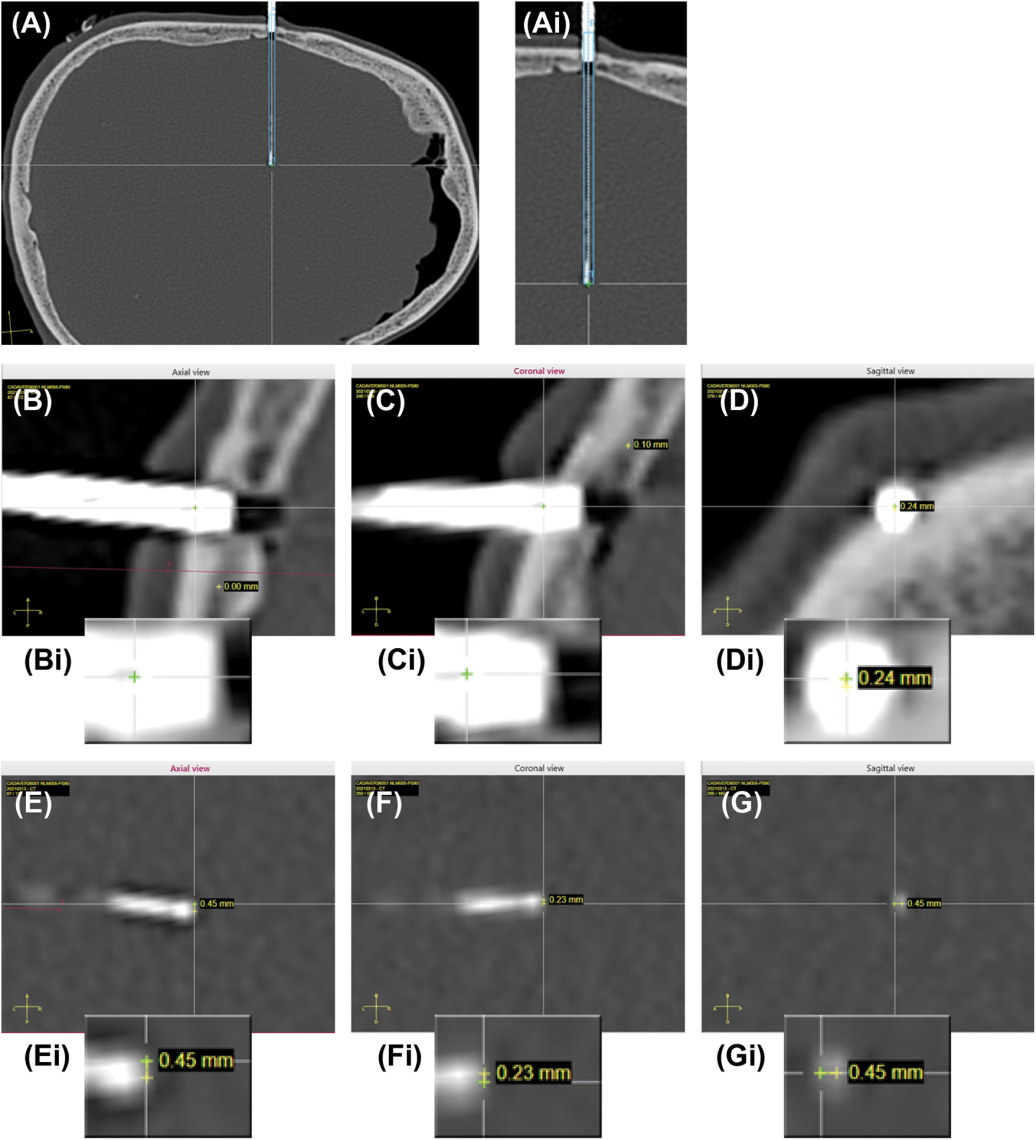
Planned and actual trajectories. (A) CT scan image of an actual implanted electrode is co-registered with the planned trajectory (dotted blue lines) and expected electrode location (solid blue line). Ai. Higher magnification of the actual implanted electrode and planned trajectory. (B–D) Example of EPE measurements in all 3 planes (axial, sagittal, coronal). Bi-Di Insets showing higher magnification images of the entry points. Yellow cross is planned trajectory, green cross is actual trajectory. The difference is the error, shown for each plane. (E–G) Example of TPE measurements in all 3 planes (axial, sagittal, coronal). Ei-Gi Insets showing high magnification images of the target points. Yellow cross is planned trajectory, green cross is actual trajectory. The difference is the error, shown for each plane.
